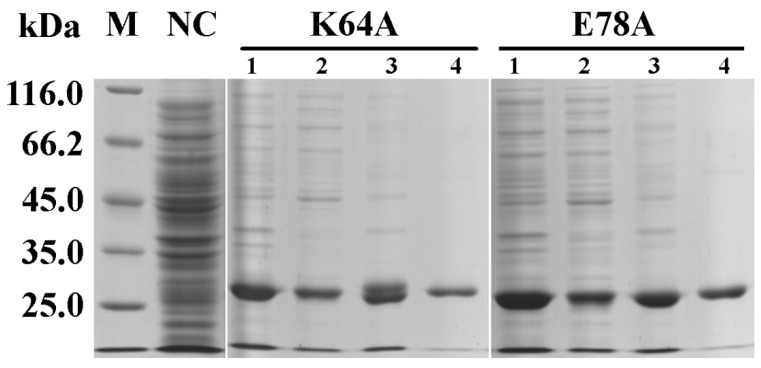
Analysis of the expression and purification of mutant proteins by SDS-PAGE. Lane M: Protein marker; Lane NC: Negative control (bacteria containing modified pET30 plasmids); Lane 1: Bacteria containing recombinant plasmids after being induced by IPTG; Lane 2: Supernatant of cell lysate by ultrasound; Lane 3: Precipitate of cell lysate by ultrasound; and Lane 4: Purified recombinant mutant proteins.
